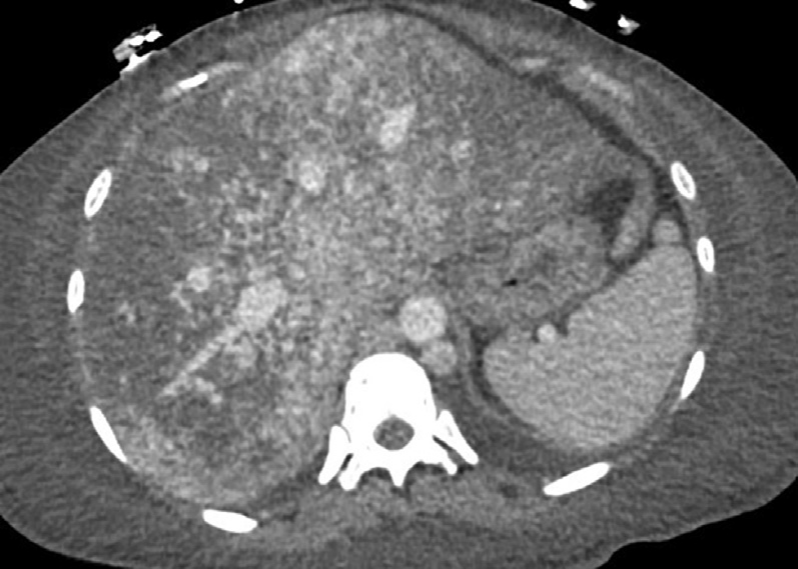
3-Phase Liver Computed Tomography Innumerable liver venous malformations visualized with computed tomography.
